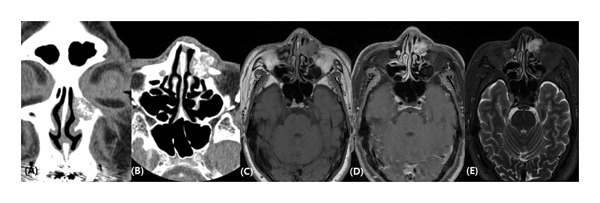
Coronal (A) and axial (B) CT showed a round honeycomb‐appearance mass in the left nasal bone compressing the nasolacrimal duct. T1‐weighted (C), contrast‐enhanced T1‐weighted (D), and T2‐weighted (E) MRI images demonstrated a heterogenous ovoid mass measuring 21 × 14 mm, protruding into the nasal cavity.
